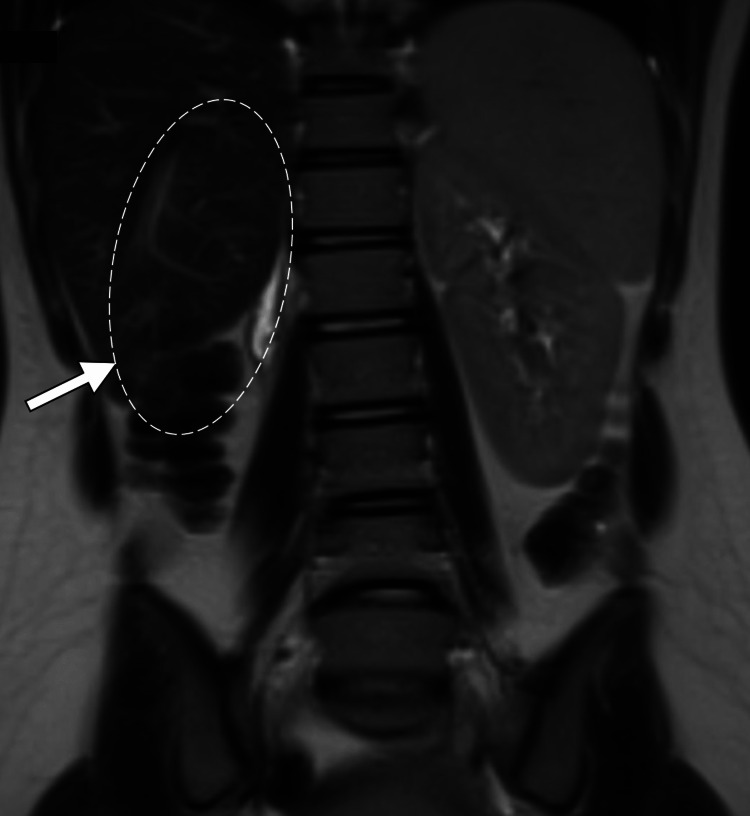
Coronal T2-weighted MR image showing right renal agenesis (arrow). MR: magnetic resonance
